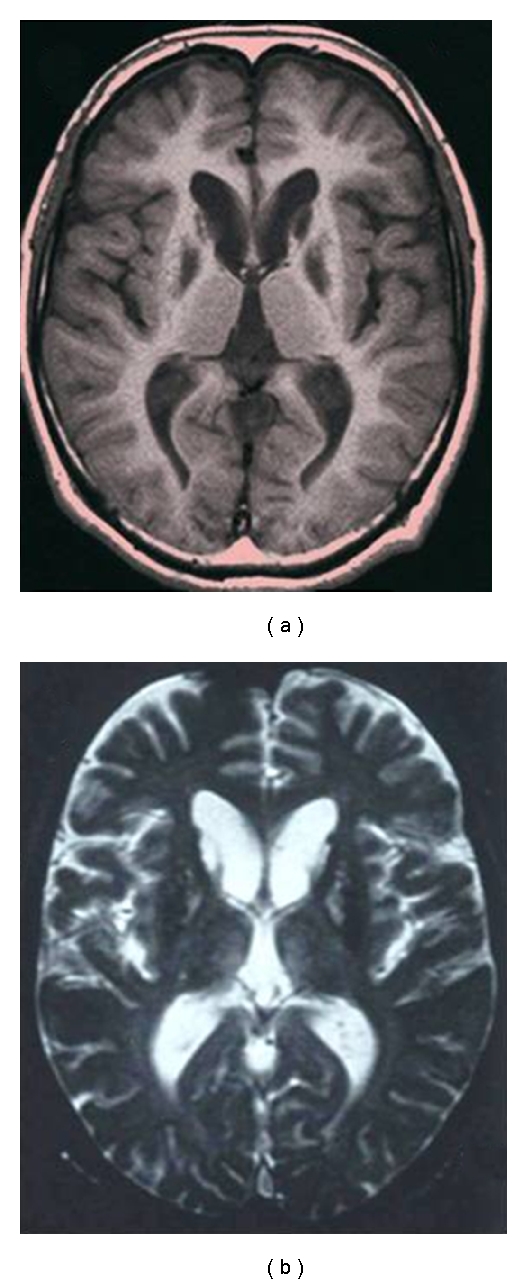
Axial section at the level of the basal ganglia in the patient at 35 years of age. (a) A T1-weighted image (TR 400 msec/ TE 14 msec) shows symmetrical hypointense signals in the head of the caudate nucleus and globus pallidus. (b) A T2-weighted image (TR 800 msec/TE 30 msec) shows hypointense changes in the lenticular nucleus. Hyperintense signals can be observed in the putamen and the head of the caudate nucleus.
